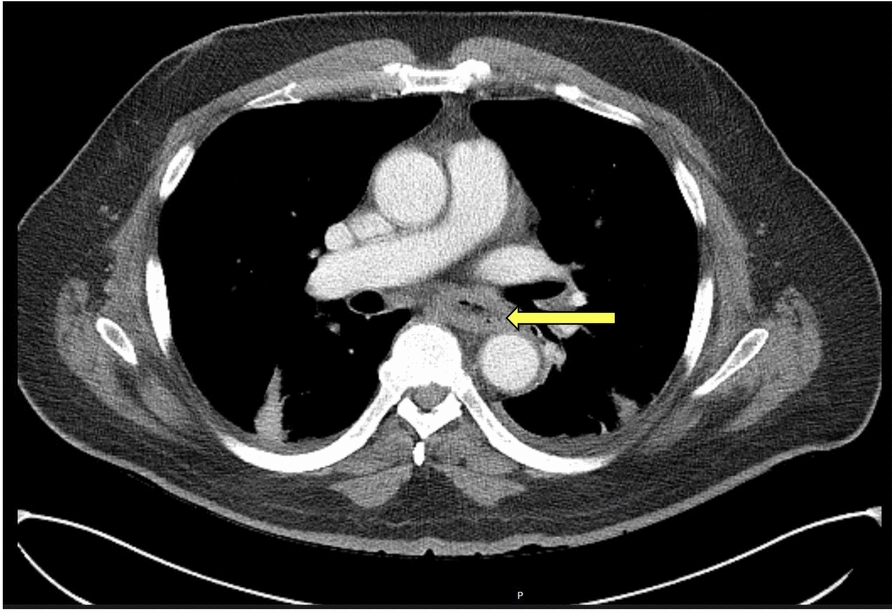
Computed tomography scan with intravenous contrast revealing a thick-walled esophagus (arrow) suggestive of esophagitis along with debris and fluid within the lumen, without any evidence of perforation
